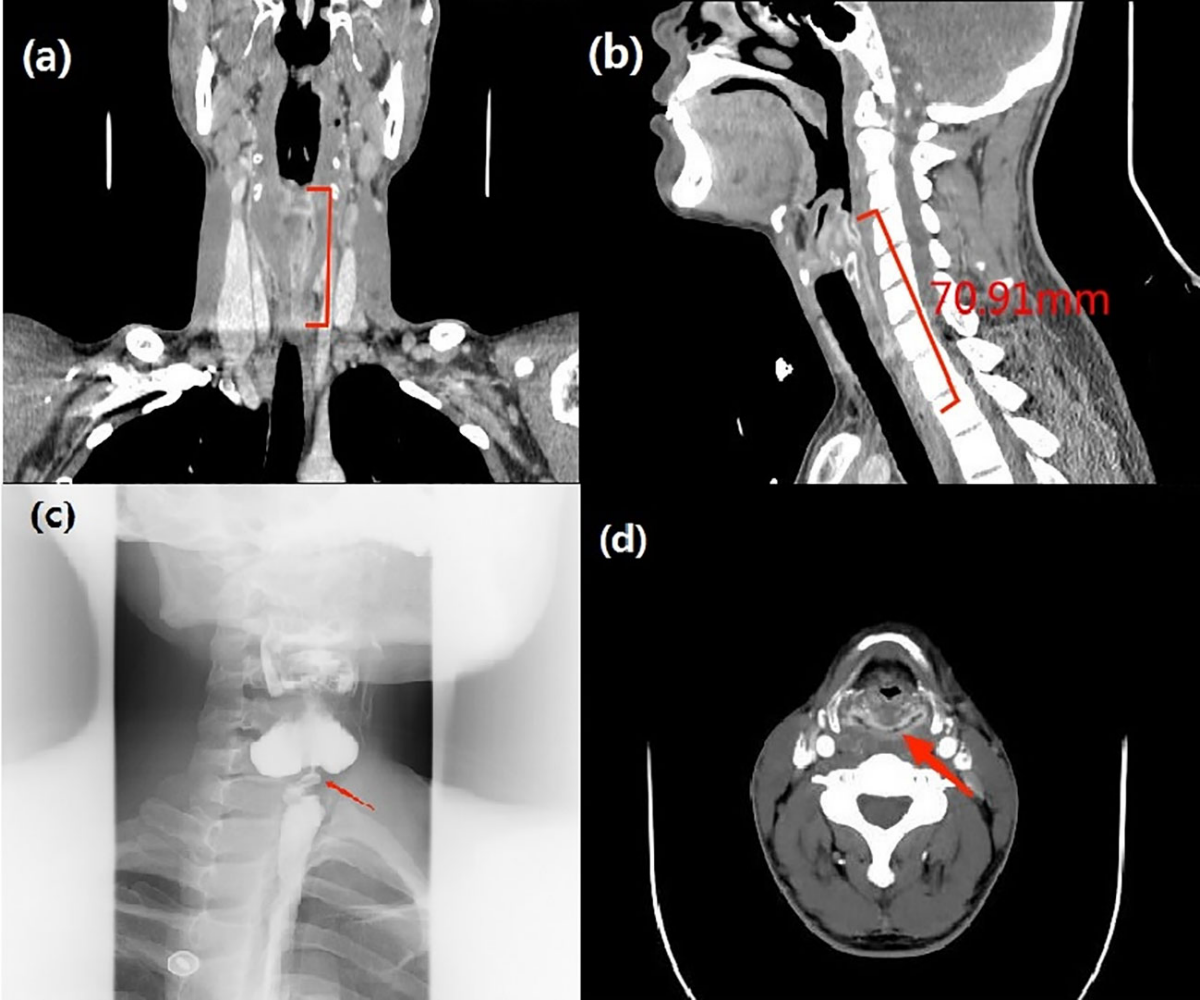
(A, B) Neck-enhanced CT: (sagittal and coronal): The mucosa of the upper esophagus was thickened and enhanced to a length of 71 mm. (C) Esophageal barium swallowing test: severe stenosis of the horizontal lumen of the C6-C7 vertebral body in the upper esophagus. (D) Neck-enhanced CT: (axial): The mucosa of the posterior area of the cricoarytenoid joint showed annular enhancement.
